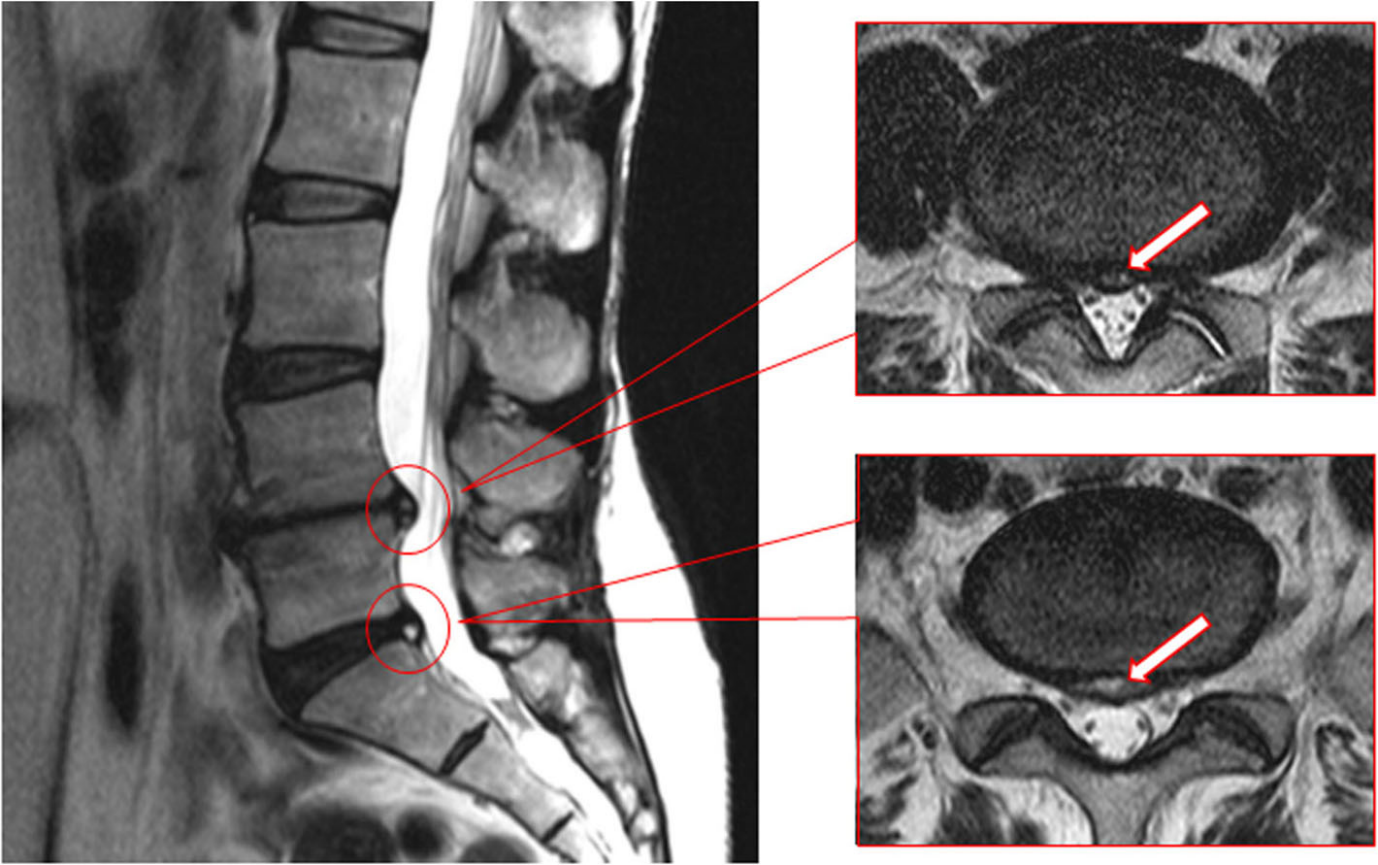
T2-weighted fast spin-echo sagittal and axial MR images demonstrating mid-posterior HIZs at the L4-5 and L5-S1 levels
